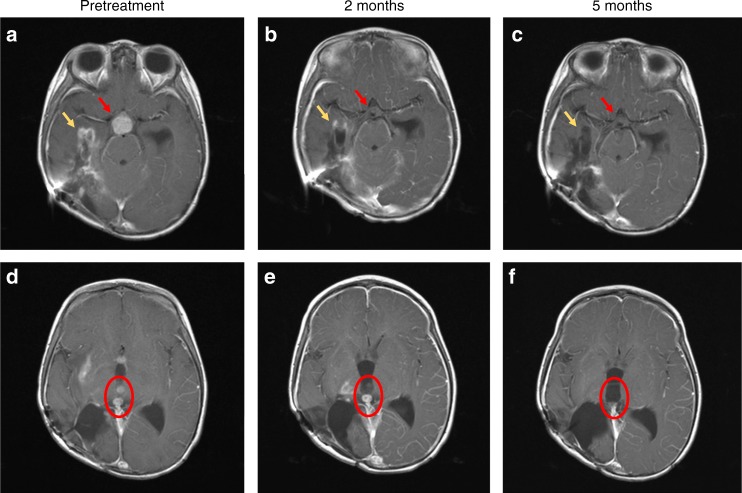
T1-weighted brain magnetic resonance imaging (MRI) with contrast images shown pre-treatment a, d, after 2 months of treatment b, e and after 5 months of treatment c, f. The contrast enhancing suprasellar mass (red arrows, a), had resolved after 2 months of treatment b, with sustained response at 5 months (c). Tumour bed enhancement (yellow arrows) improved at 2 months (b), and near complete at 5 months c. Examples of two contrast enhancing intraventricular lesions (red circle) pre-treatment d, improved after 2 months e and with complete resolution at 5 months f. The contrasting enhancing disease in the right thalamus are also visible in e, and had completely resolved after 5 months of treatment f
